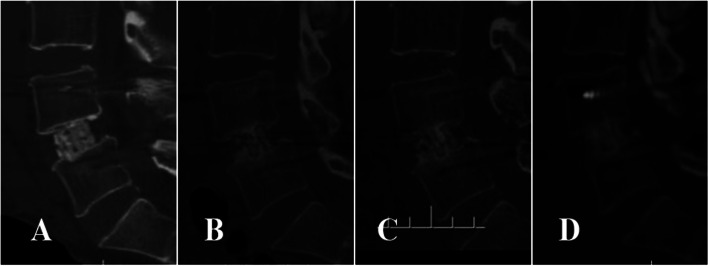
A series of post-operative computed tomography (CT) images of a 52-year-old female patient with L4–5 spinal stenosis reflecting the fusion progress over time. A Post-operative CT scan showing the fusion area; B the fusion grade of 3 at postoperative 6 months; C fusion grade of 4 at postoperative 12 months; and (D) fusion grade of 5 at the last follow-up
